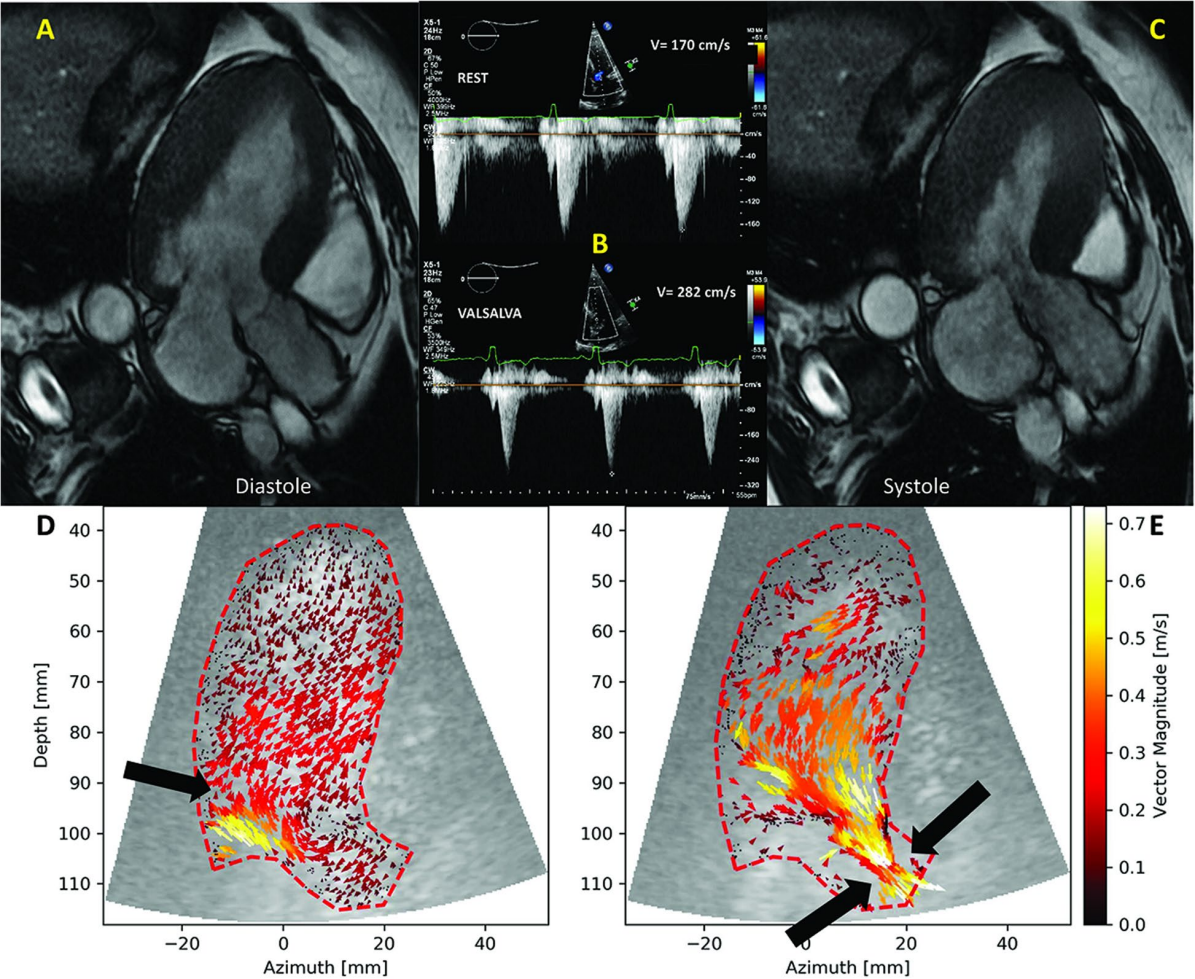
Patient 1. A, C: CMR in diastole and systole, highly suggestive for a hypertrophic cardiomyopathy; flow acceleration in the LVOT with a tendency to luminal obliteration in mid-systole; B: CW Doppler of the LVOT at rest (upper) and during Valsalva manoeuver (lower).The high-velocity flow could also be detected by careful interrogation with continuous-wave Doppler. D: HFR echoPIV in early diastole. Early diastolic inflow (black arrow) had higher velocity and an vertical direction (Additional file 5), and echoPIV revealed the lacking of the mid-LV vortex that normally appears in healthy individuals (Additional file 1), probably as a result of diastolic dysfunction.; E: HFR echoPIV in mid-systole. LVOT flow acceleration and narrowing (black arrows)
